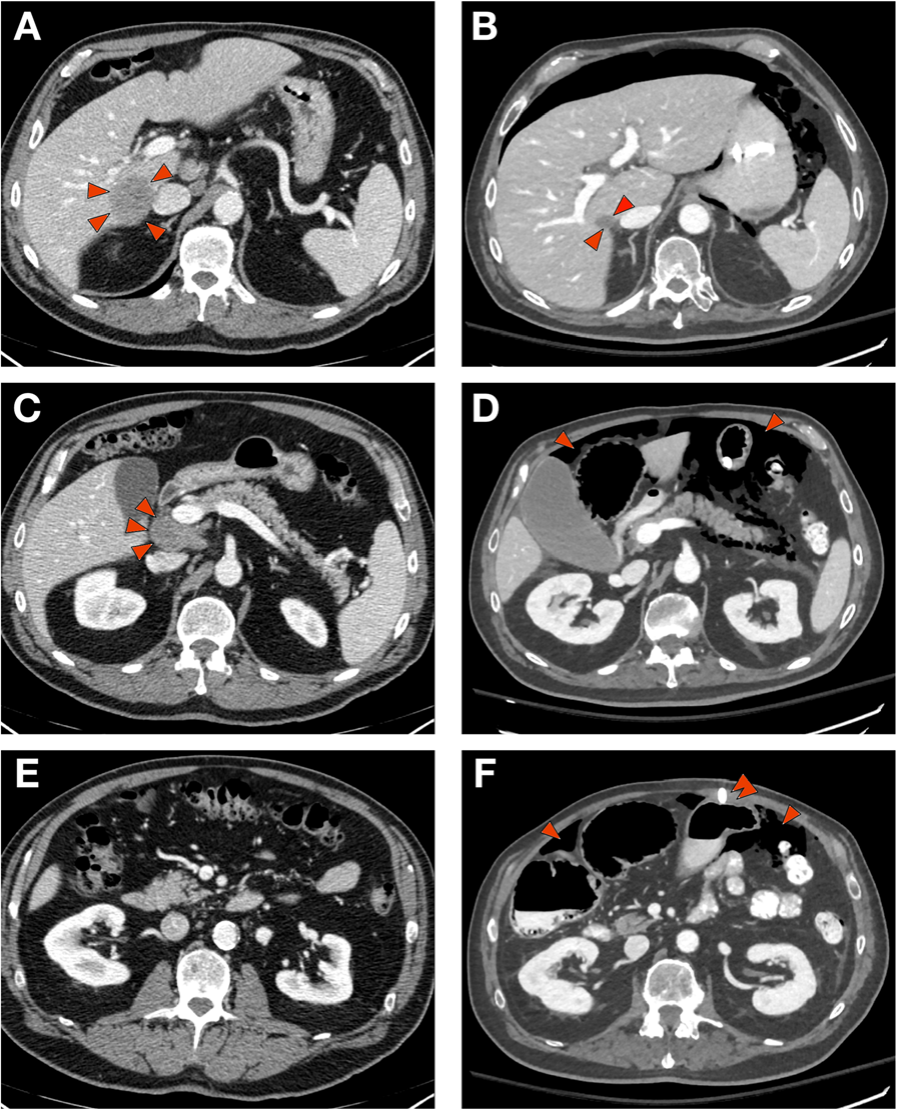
CT images obtained following the administration of IV contrast prior to (panels a, c, and e) and 51 day following treatment (panels b, d, f) with ipilimumab and nivolumab. The pretreatment scans show a 4.2 × 4.0 cm lesion within the right lobe of the liver lateral to the inferior vena cava (a, the lesion is identified by arrowheads), as well as peripancreatic retroperitoneal adenopathy (c, identified by arrowheads). Following treatment, the lesion within the liver has decreased to 2.0 × 1.6 cm (b, identified by arrowheads) and the retroperitoneal adenopathy has resolved (d). Dilated loops of large bowel as well as peritoneal air is present (panels d and f, identified by arrowheads) as well as a percutaneous gastrostomy tube (panel d, double arrowhead)
